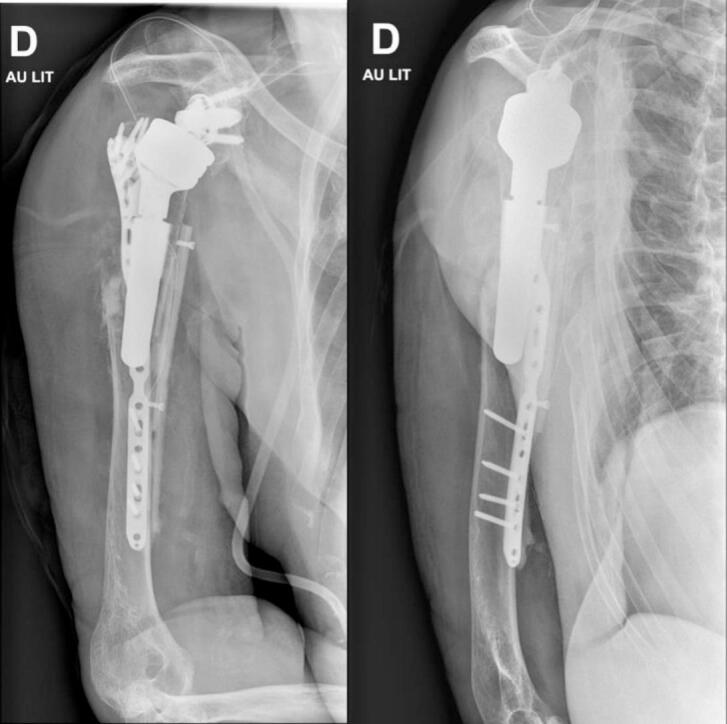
Postoperative X-rays after revision ORIF for periprosthetic humeral fracture.
